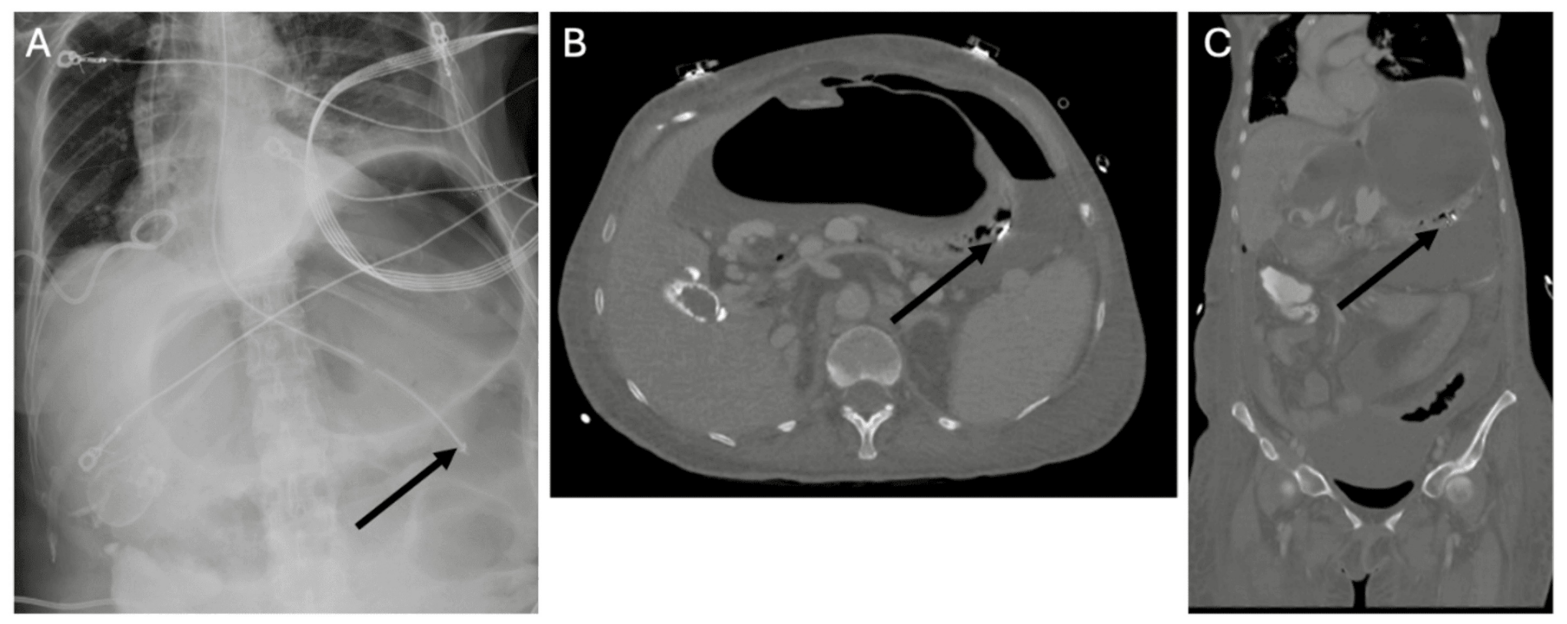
Nasogastric Tube Malposition With Suspected Gastric Wall Erosion and Perforation Imaging of a malpositioned nasogastric (NG) tube with evidence of complication. (A) Chest radiograph shows the NG tube coursing below the diaphragm with the distal tip projecting over the left upper quadrant (arrow) seemingly in the correct space. (B, C) Axial and coronal non-contrast CT images demonstrate the tube tip abutting and extending beyond the gastric wall, with associated free intraperitoneal air and fluid (arrows), concerning for gastric wall erosion and perforation. Prompt recognition is critical, as continued feeding through a malpositioned tube may exacerbate peritonitis and sepsis.
